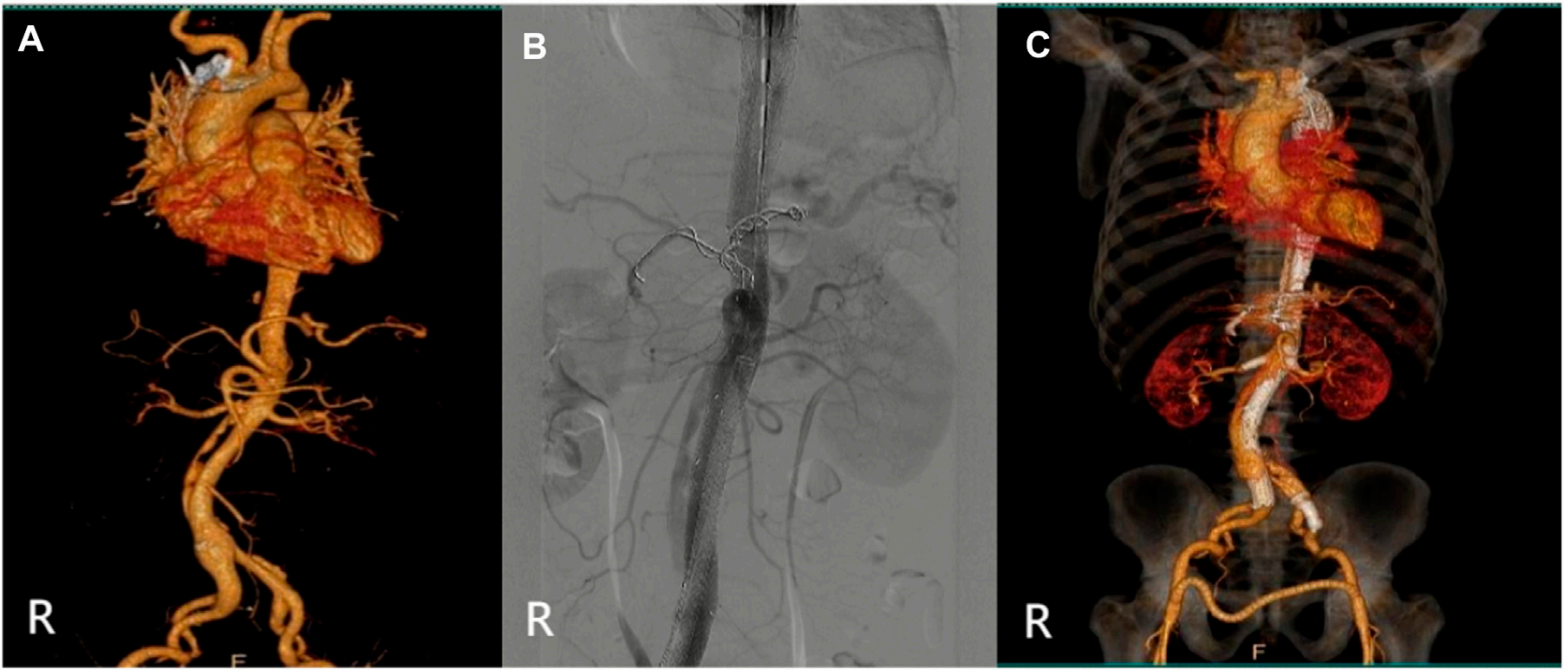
(Case 4) (A) Preoperative CTA. (B) Intraoperative imaging. (C) CTA (1st week).
